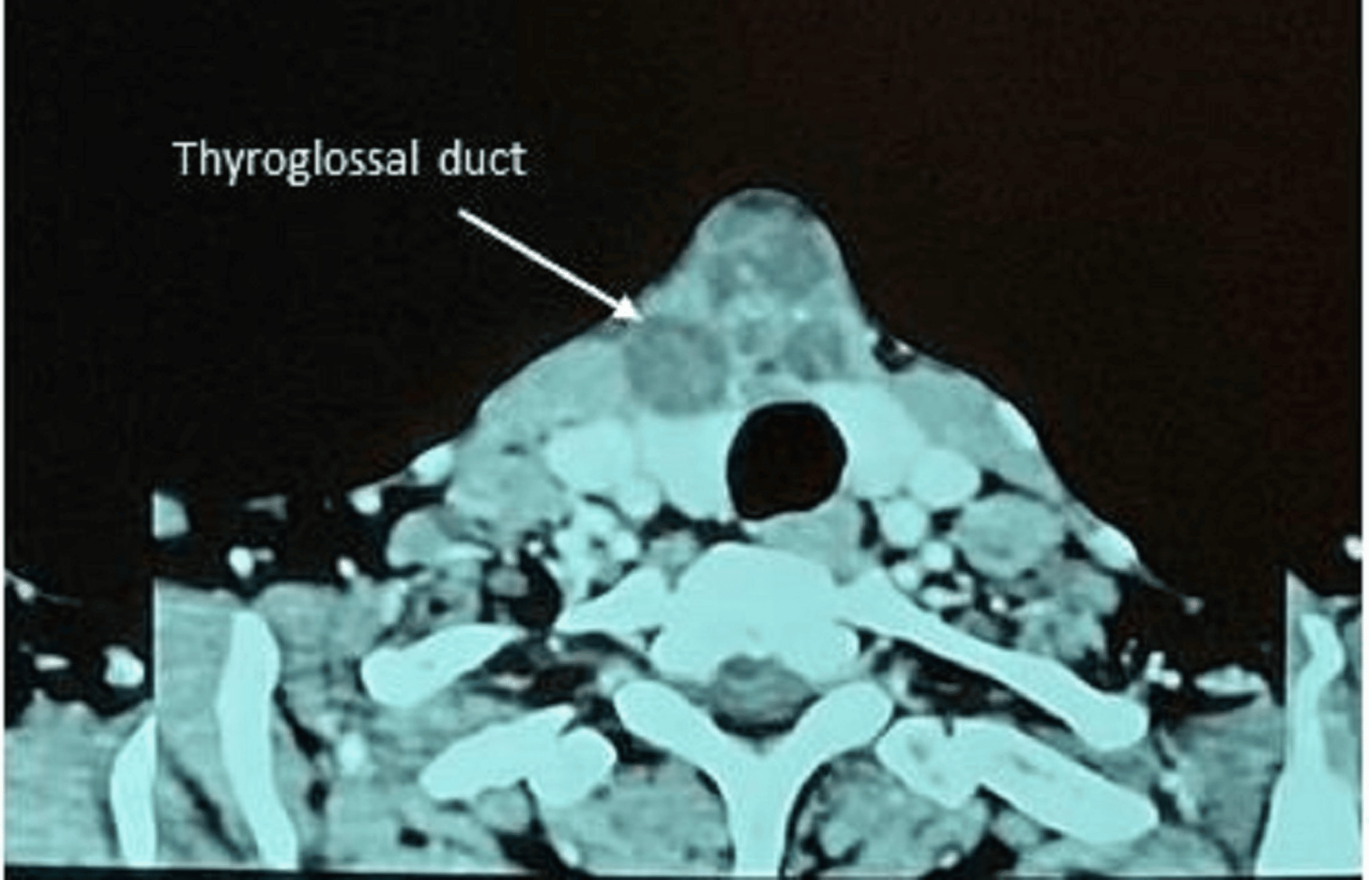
CT scan images (axial section)
